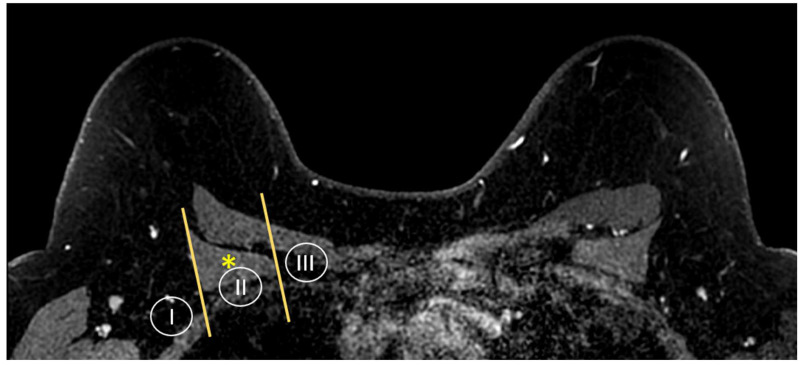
Anatomy of the axillary nodal stations on axial T1W fat-suppressed post-contrast breast MRI. Levels are defined by the relationship of the lymph node to the pectoralis minor muscle (asterisk).
